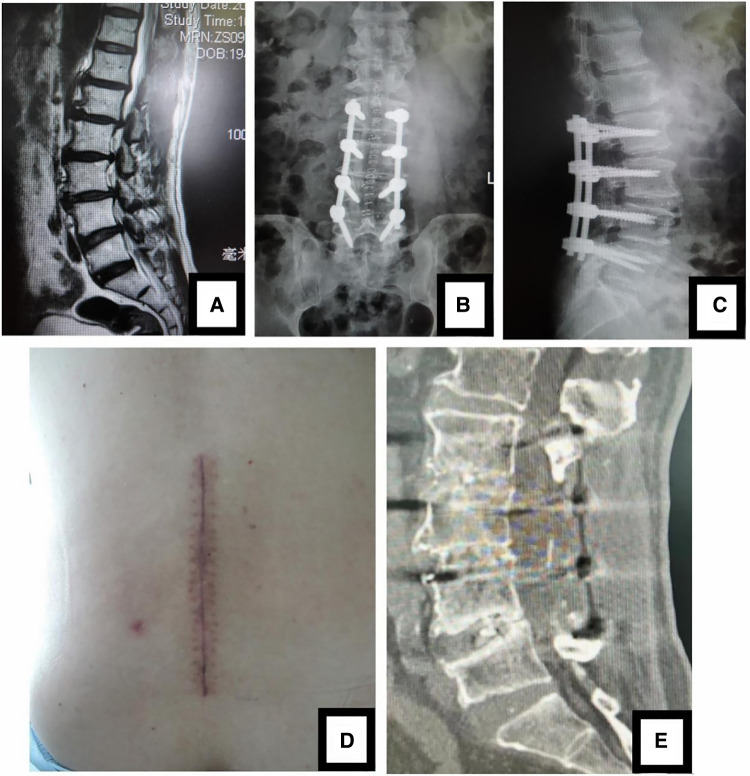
(A) sagittal MR images showed 3-level LDD (L2-L5) in a 69-year-old woman. Open TLIF was performed and (B, C) postoperative x-ray confirmed that the position of pedicle screws and cages was good. (D) The picture was postoperative incision. (E) CT scan image at 5-year follow-up showed that the neurologic decompression was complete and fusion of grade I or II was achieved.
